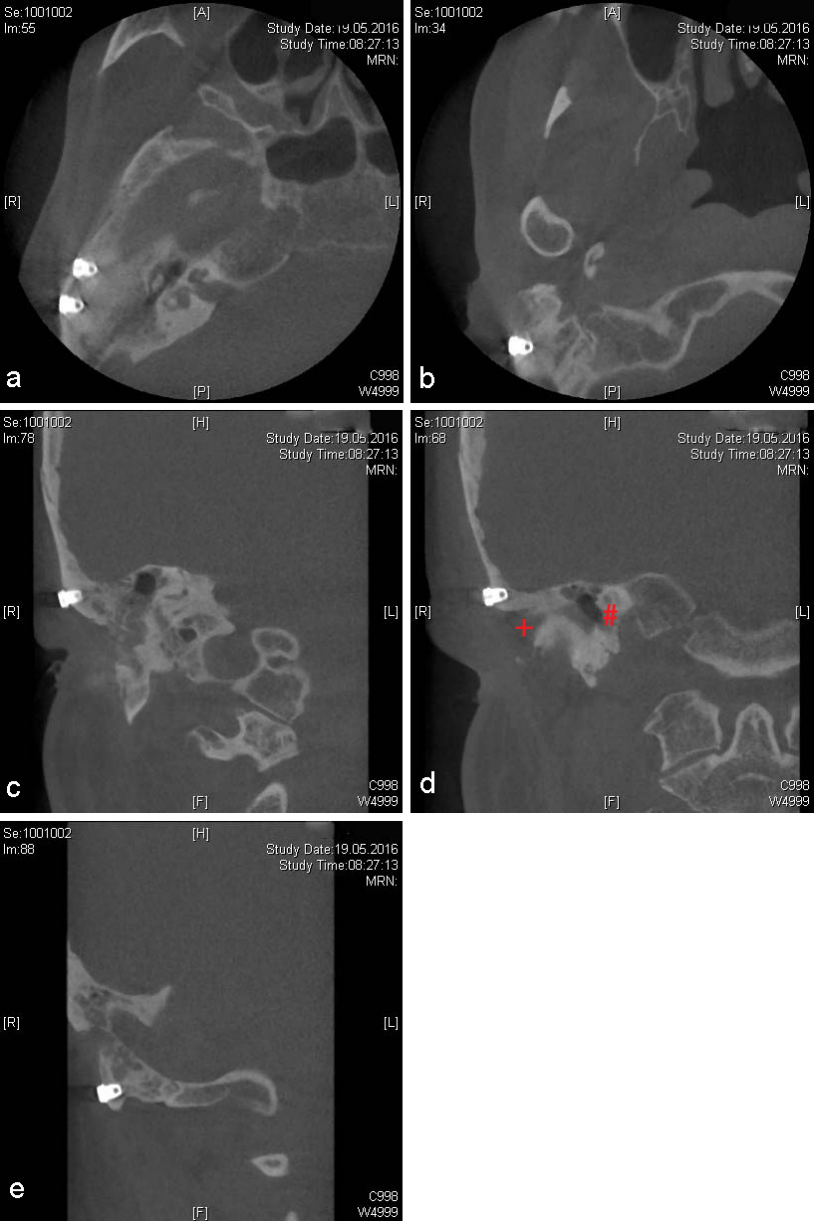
Postoperative CBCT-imaging. a: Axial section. Both cranial implant positions. b: Axial section. Caudal implant position. c: Coronal section. First cranial implant position. d: Coronal section. Second cranial implant position, red plus marking the external meatus acusticus, and red diamond indicating the cochlea. e: Coronal section. Caudal implant position.
